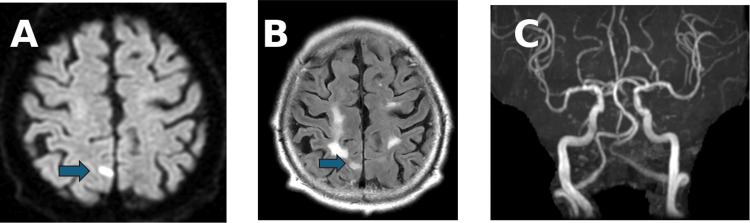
Brain MRI and angiographic findings. (A) Diffusion-weighted imaging demonstrating acute infarction in the right parietal lobe (arrow). (B) Fluid-attenuated inversion recovery imaging showing corresponding hyperintensity in the same region (arrow). (C) Magnetic resonance angiography revealing no significant large-vessel occlusion.
